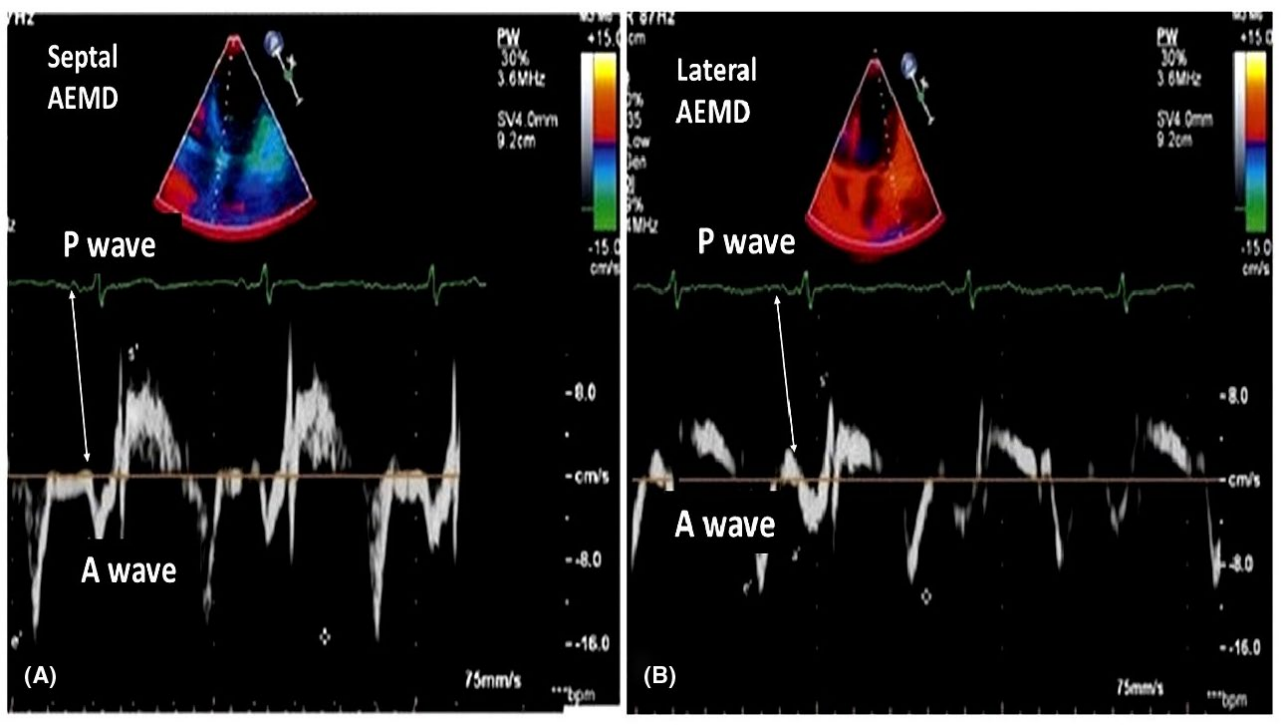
Image of echocardiographic doppler signals using tissue doppler imaging in apical four‐chamber view showing an example of atrial electromechanical delay time measurement at the septal and lateral positions of the mitral annular plane
